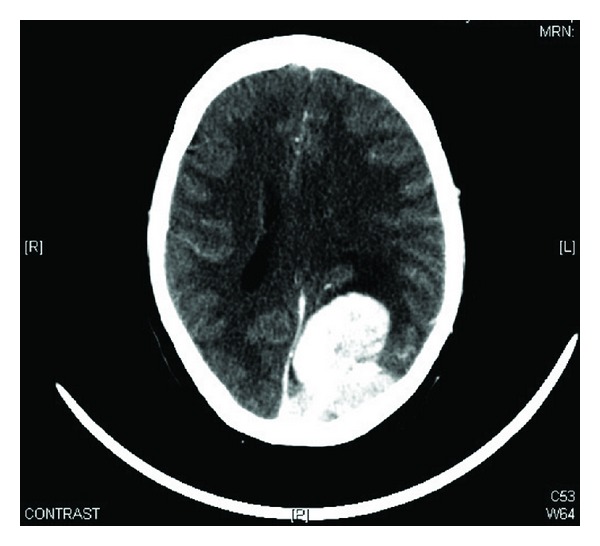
Computed tomography of brain showing multiple intra- and extracranial enhancing lesions at left occipital, left parietal subdura (5.6 × 3.5 cm) with midline shift and vasogenic edema, and right cerebellum.
